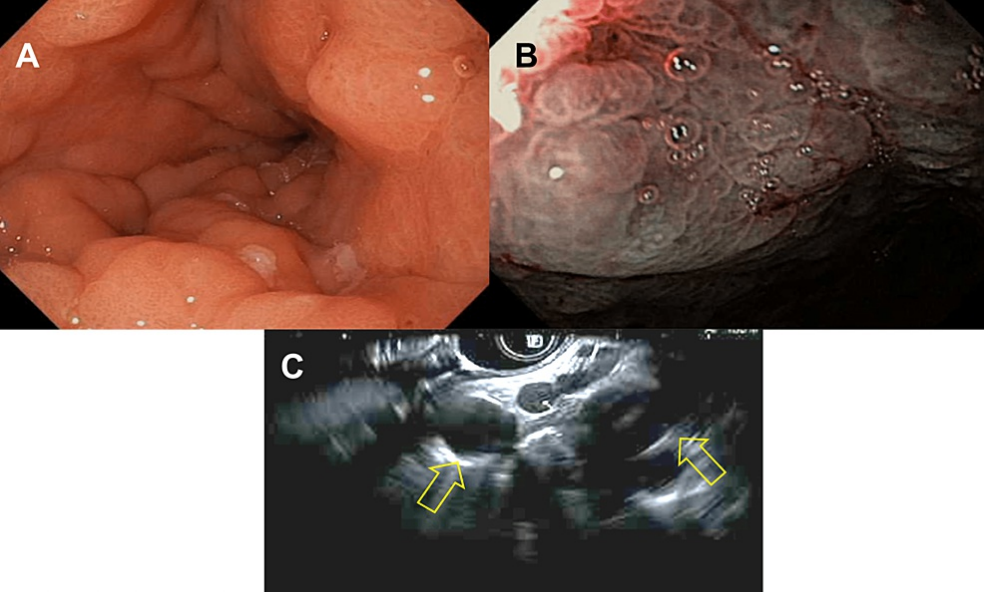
Endoscopic gross images and EUS A: Endoscopic longitudinal view of the antrum; B: Narrow-band imaging view of the distorted gastric mucosa; C: The EUS view identifying multiple lymph nodes EUS: Endoscopic ultrasound
